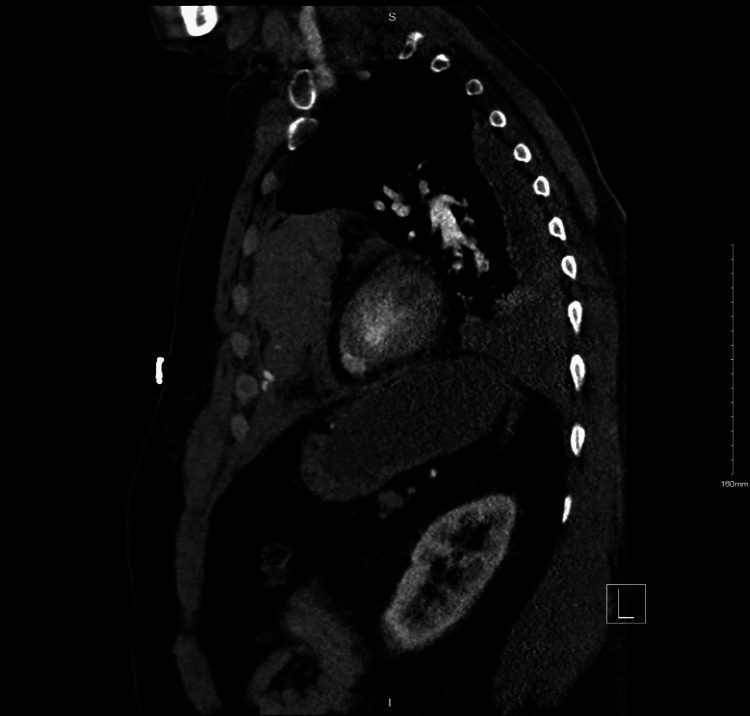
Sagittal view of the computed tomography angiography of the anterior mediastinal hematoma described
